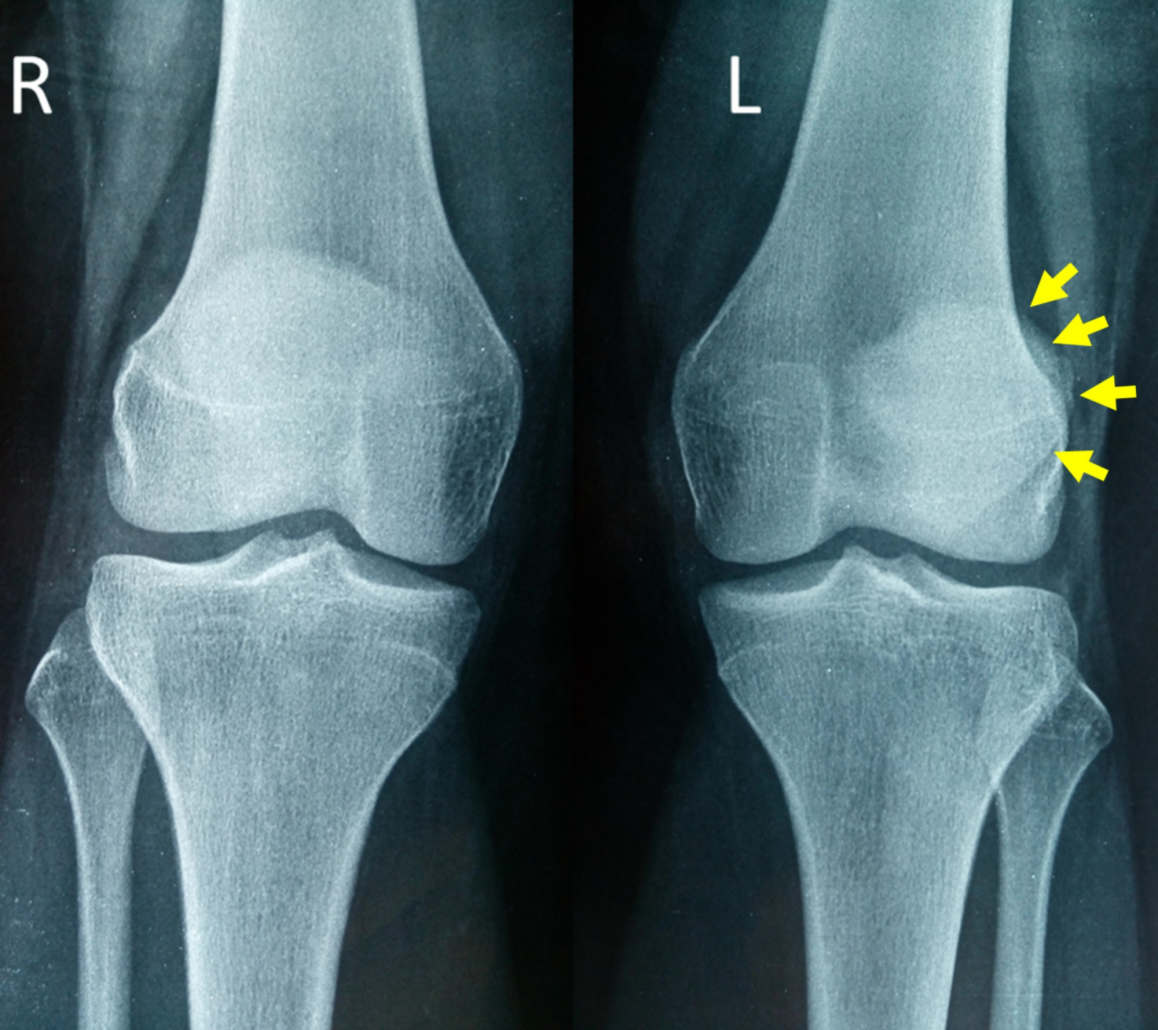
Anteroposterior X-ray of both knees in the standing position on patient admission Arrows indicate lateral subluxation of the left patella
